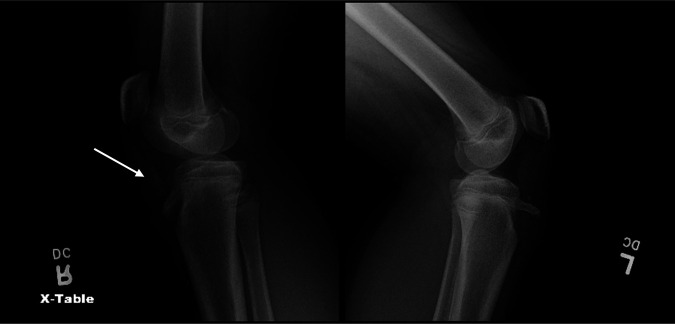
Radiographs of the right and left knees that reveal bilateral tibial tubercle fractures. The right knee reveals an ossific fragment proximal to the tibial tubercle (arrow).
